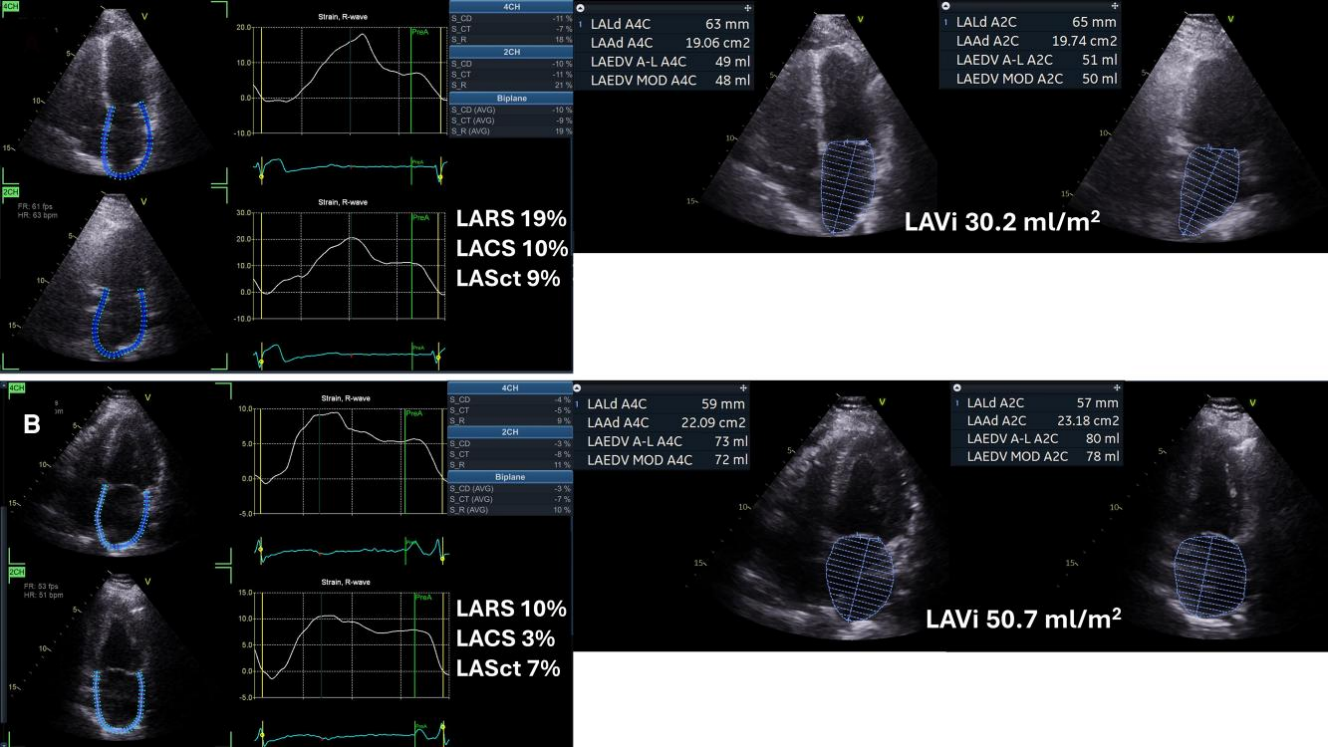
Different stages of atrial cardiomyopathy on echocardiographic imaging. Upper panel (*A*)—early AtCM with moderately reduced LA strain and normal LA volume. Lower panel (*B*)—advanced AtCM with severly reduced LA strain and LA dilatation, clinically consistent with atrial failure
